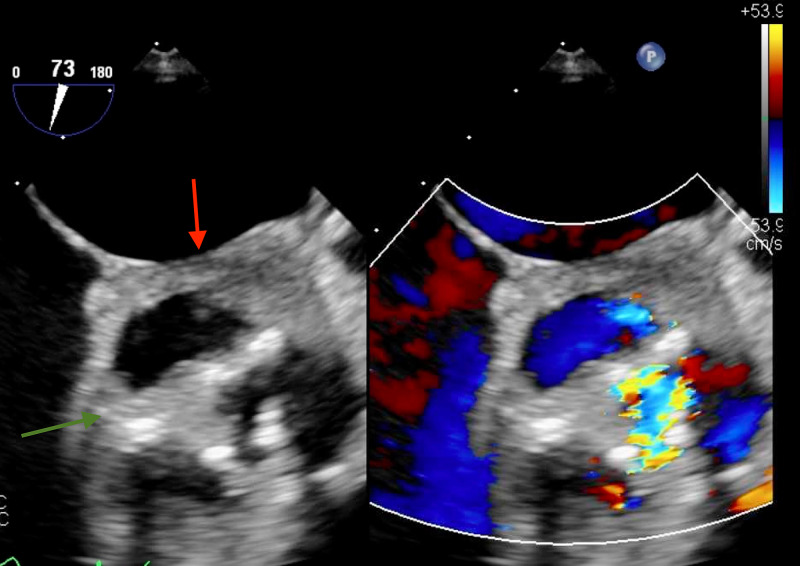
TEE of the aortic valve showing vegetations (green arrow) along with surrounding lucency suggestive of abscess formation (red arrow). TEE, transesophageal echocardiogram
